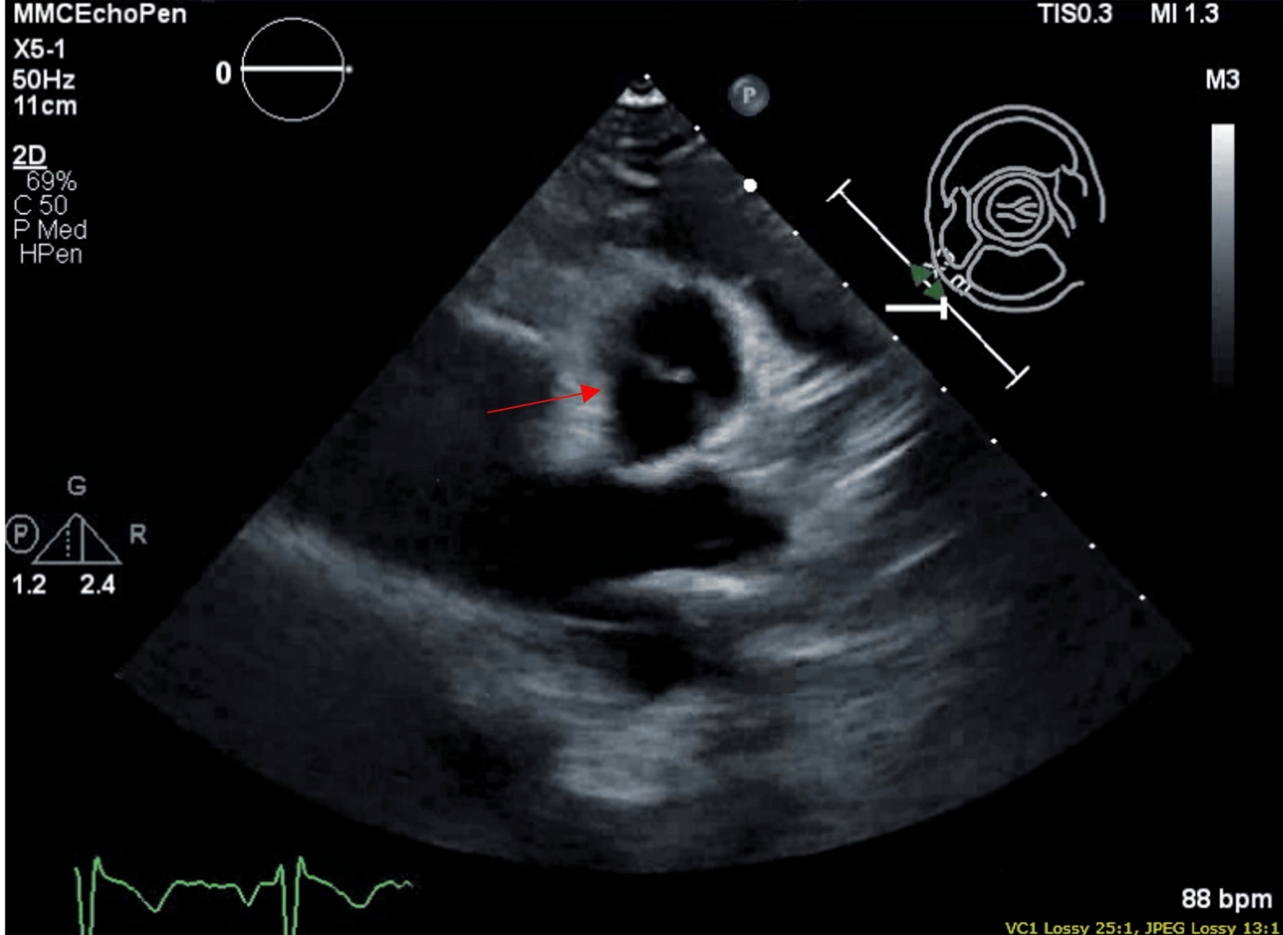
TTE showing bicuspid aortic valve with no evidence of vegetation TTE: transthoracic echocardiogram
